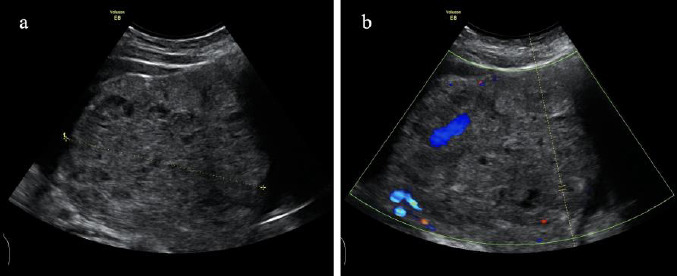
Transabdominal ultrasound image description. (a) Two-dimensional ultrasound revealed a large heterogeneous solid mass in the pelvic cavity. (b) CDFI showed blood flow signals both within and around the mass.
